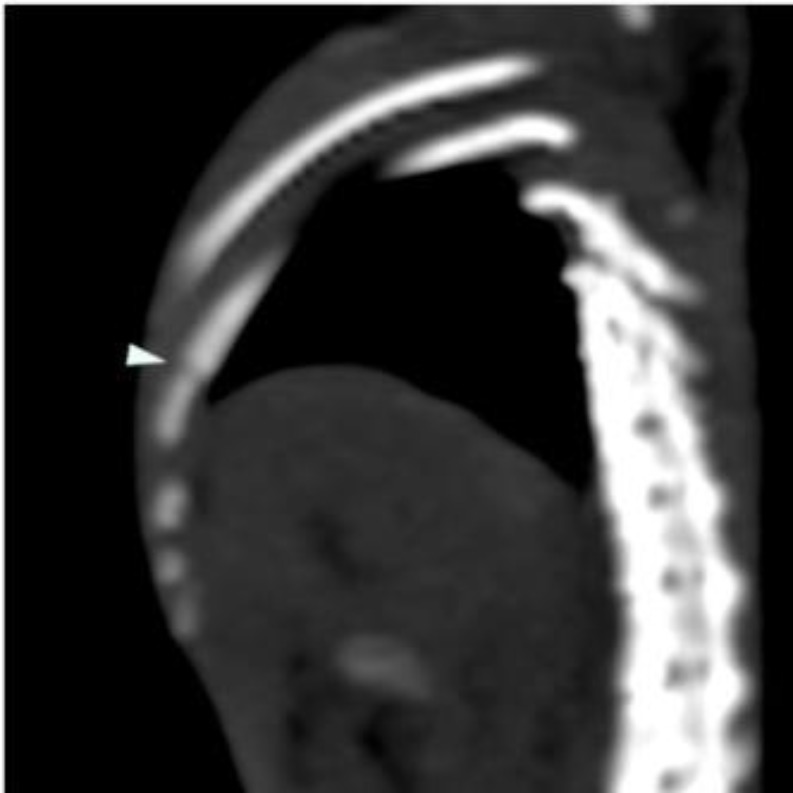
In thorax CT the rib fracture is indicated by the white arrow
